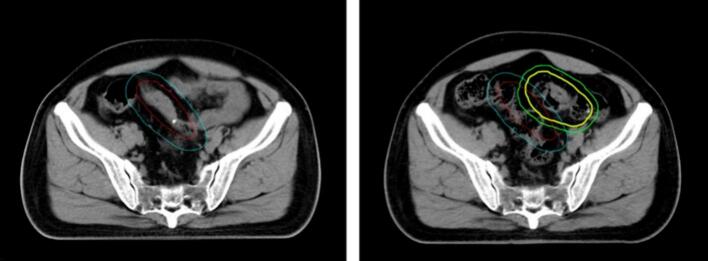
Representative transverse CT slice from the case with the lowest DSC for the target volume. The left panel shows the reference CT, and the right panel shows the corresponding online CT of the last fraction. Red and blue contours represent the GTV and PTV on the reference CT, respectively, while yellow and green contours represent the GTV and PTV on the online CT.
